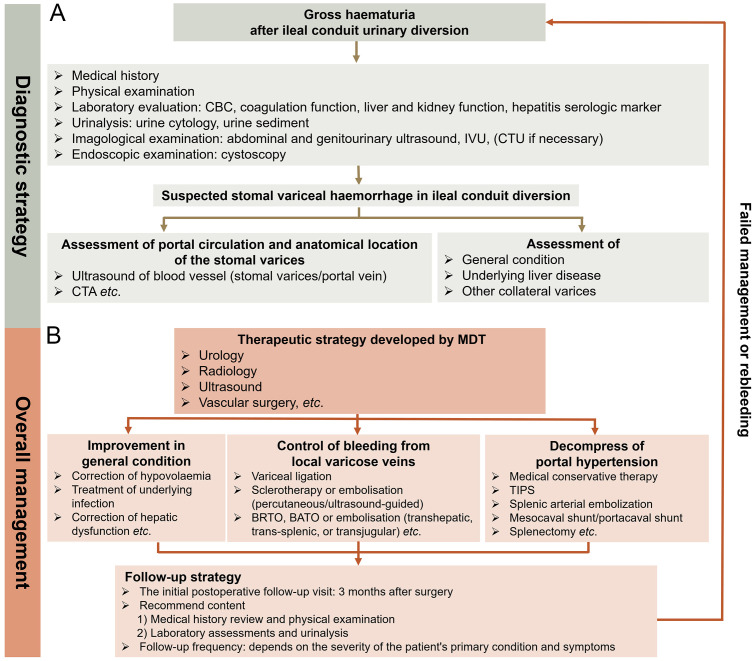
Diagnostic strategy and comprehensive management of stomal variceal haemorrhage in ileal conduit diversion. (A) Diagnostic strategy. Accurate diagnosis of stomal variceal haemorrhage involves ruling out other common conditions (especially genitourinary cancer and urinary obstruction/calculi) that may lead to haematuria and identifying potential portal hypertension. If ultrasound, cystoscopy, and IVU fail to pinpoint the cause of haematuria, a CTU examination is recommended. Utilizing reconstructed CTA provides precise visualization of the course of ileal varices, allowing urologists and radiologists to plan therapeutic interventions effectively. In cases indicating signs of portal hypertension, it is crucial to investigate the presence of varices in collateral venous pathways of the portal system, particularly oesophageal and gastric varices. (B) Comprehensive management. It is advisable for an MDT to collaborate in developing treatment strategy. The MDT should comprise experts in urology, radiology, ultrasound, and vascular surgery, with potential involvement in general surgery and hepatology as needed. Therapeutic decisions should be based on understanding the anatomical location of stomal varices, the severity of underlying liver disease, and a thorough assessment of the patient. Local therapeutic options such as cyanoacrylate injection, thrombin injection, and variceal band ligation, along with interventional radiological procedures like BRTO, BATO, and embolization (transhepatic, transjugular, and trans-splenic), are considered first-line treatments. Decompressive therapy for portal hypertension hinges on the anatomy and patency of the portal vascular circulation and severity of portal hypertension. Portosystemic shunting surgeries or splenectomy for treating stomal varicose haemorrhage are warranted only in highly selective cases where local and interventional radiological approaches have proven ineffective or are technically unfeasible. The initial postoperative follow-up visit is advised to take place 3 months after the surgery and should include a comprehensive medical history review, physical examination, laboratory assessments, and urinalysis. The frequency of subsequent follow-ups depends on the severity of the patient’s primary condition and symptoms. Patients must be informed to promptly seek medical attention if recurrent bleeding occurs. IVU, intravenous urography; CTU, computed tomography urography; CTA, computed tomography angiography; MDT, multidisciplinary team; BRTO, balloon-occluded retrograde transvenous; BATO, balloon-occluded antegrade transvenous obliteration.
